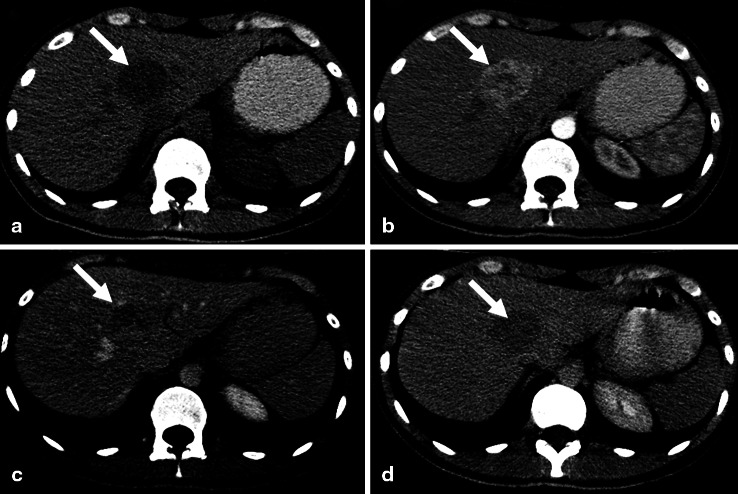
Multidetector CT, axial images. 4.4 × 3.3 cm, well defined, heterogenous, and hypodense lesion in liver segments VIII and IV upon pre-contrast scan (a); lesion demonstrated significant enhancement in arterial (b) and less enhancement in portal venous phases (c); no enhancement of the irregular area within the lesion, and definite contrast washout pattern was present in delayed phase (d)
